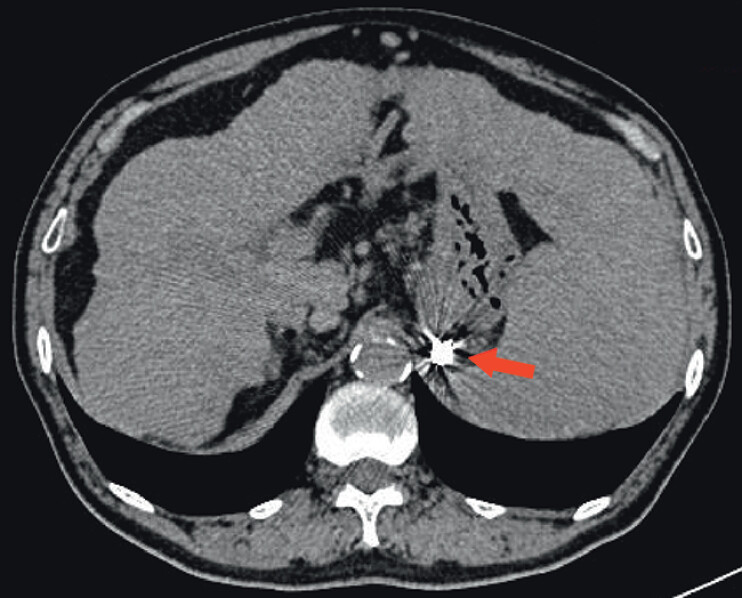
Postprocedure CT showed the coils and cyanoacrylate glue remained in situ.
